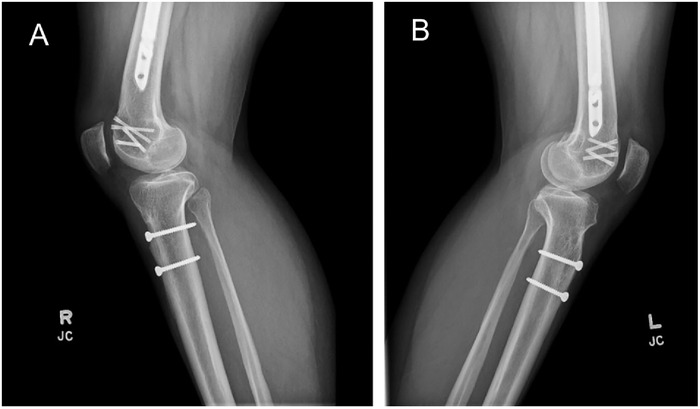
Lateral radiographs show (A) right knee 11 months postoperatively, and (B) left knee 2 years postoperatively.
